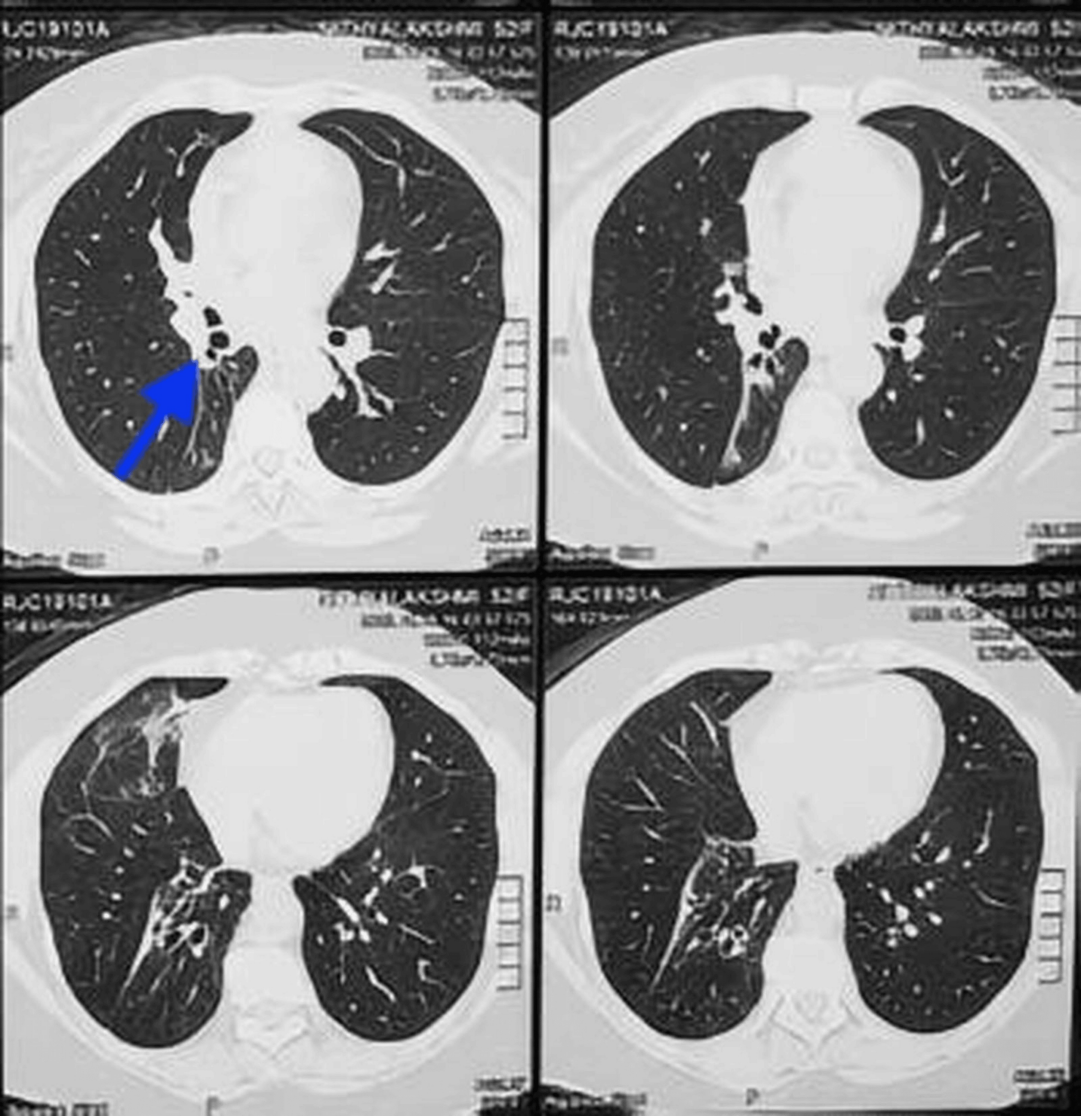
Postoperative CT images showing the re-expansion of the previously collapsed lobe (blue arrow) Axial HRCT thorax images taken post-endobronchial resection demonstrate complete re-expansion of the right lower lobe. No residual lesion or post-obstructive consolidation is seen. Lung parenchyma appears aerated and well preserved. HRCT, High-Resolution Computed Tomography
